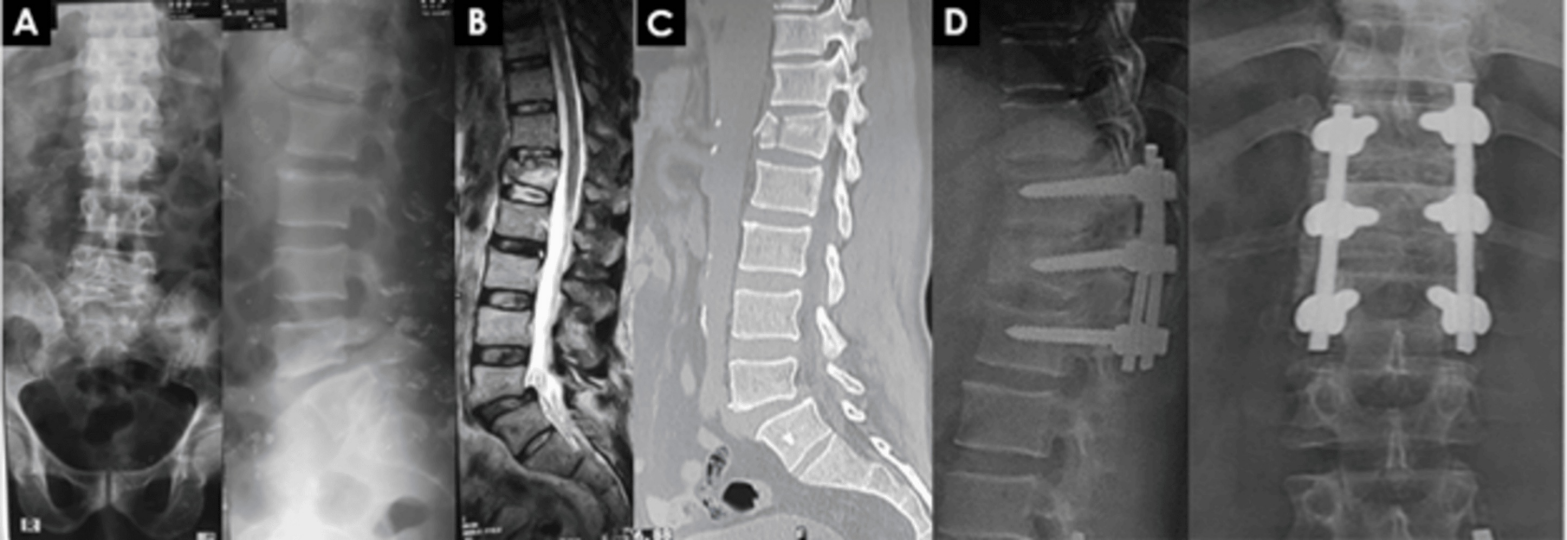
61-year-old male with burst #L1 with ASIA-C neurology, treated with short-segment posterior fixation including fractured vertebra and posterolateral fusion (Patient-1). X-ray reveals that a 61-year-old male (burst #L1 with ASIA-C neurology) who underwent short-segment posterior fixation, which included vertebral fracture and posterolateral fusion. Panels A–D report the following: A: Pre-operative X-ray, lumbo-sacral spine (AP and lateral view) B: MRI of LS spine (T2 sagittal section) C: CT scan of LS spine (sagittal section) D: Final follow-up at three years showing good fusion Image Credit: Sharif Jonayed
